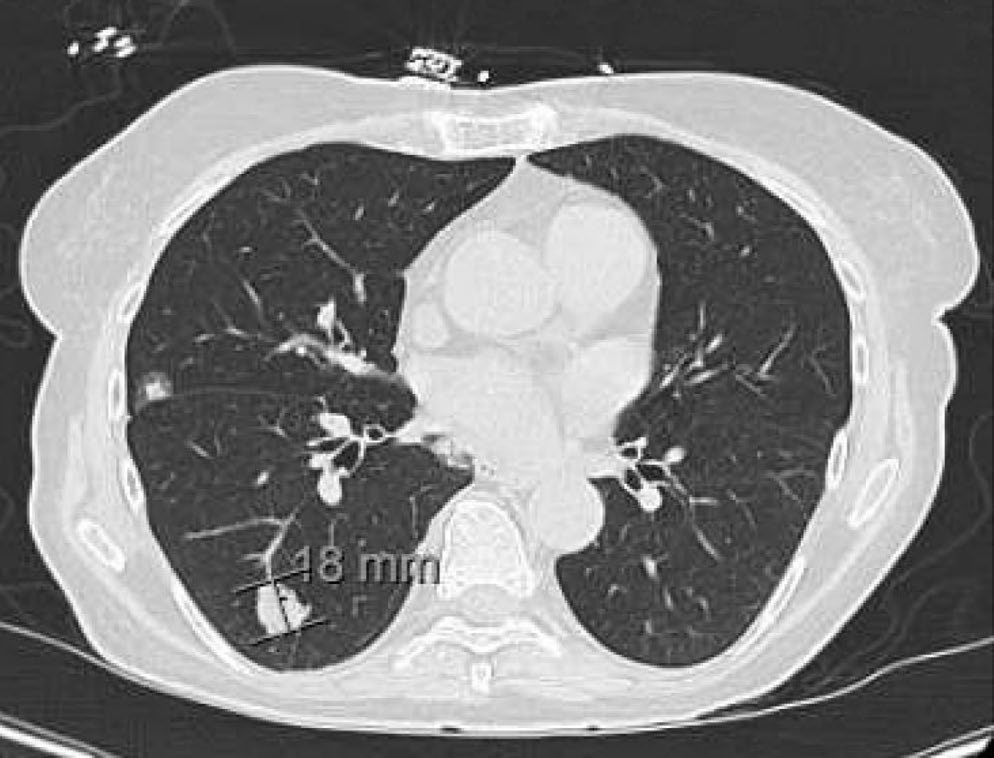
CT Chest showing pulmonary nodule suggestive of metastatic disease.
